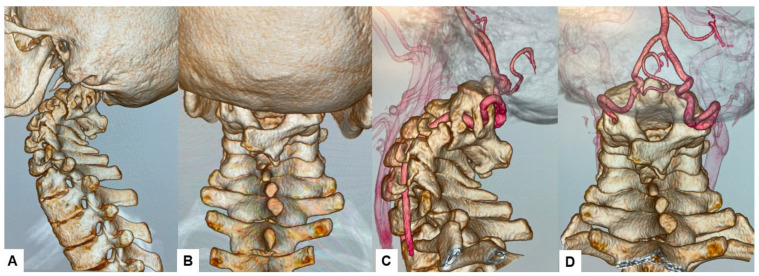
Preoperative 3D-CT and 3D-CT angiogram, (A) Lateral view 3D-CT, (B) Posterior view 3D CT, (C) Lateral view 3D-CT angiogram, (D) Posterior view 3D CT angiogram.
